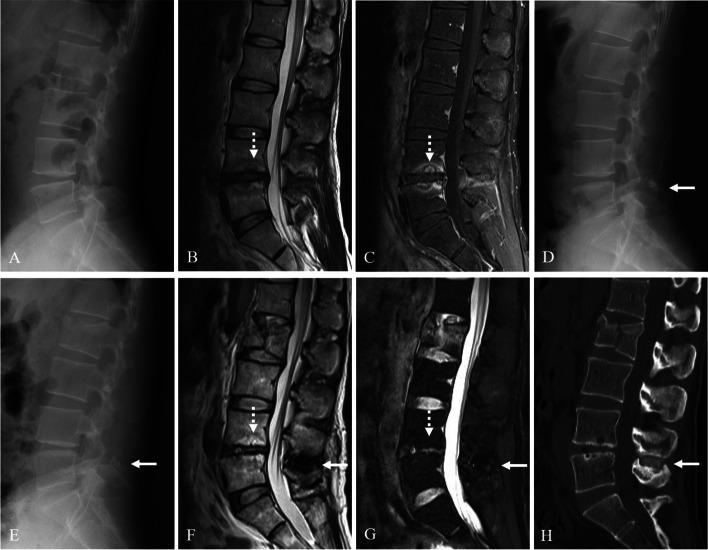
A 34-year-old woman with lumbar disc herniation (LDH) at L4–5. Lateral radiograph (A) and sagittal magnetic resonance images (B and C) showing degenerative changes and severe modic change (dotted white arrows) at L4–5. The patient underwent discectomy and DIAM implantation at L4–5 (white arrow) (D). At 11 years after discectomy and DIAM implantation at L4–5, lateral radiograph (G), sagittal magnetic resonance images (F and G) and computed tomogram (H) show well maintenance of DIAM and markedly improvement of modic changes (white arrows) at L4–5 (white arrows) without recurrence of LDH
